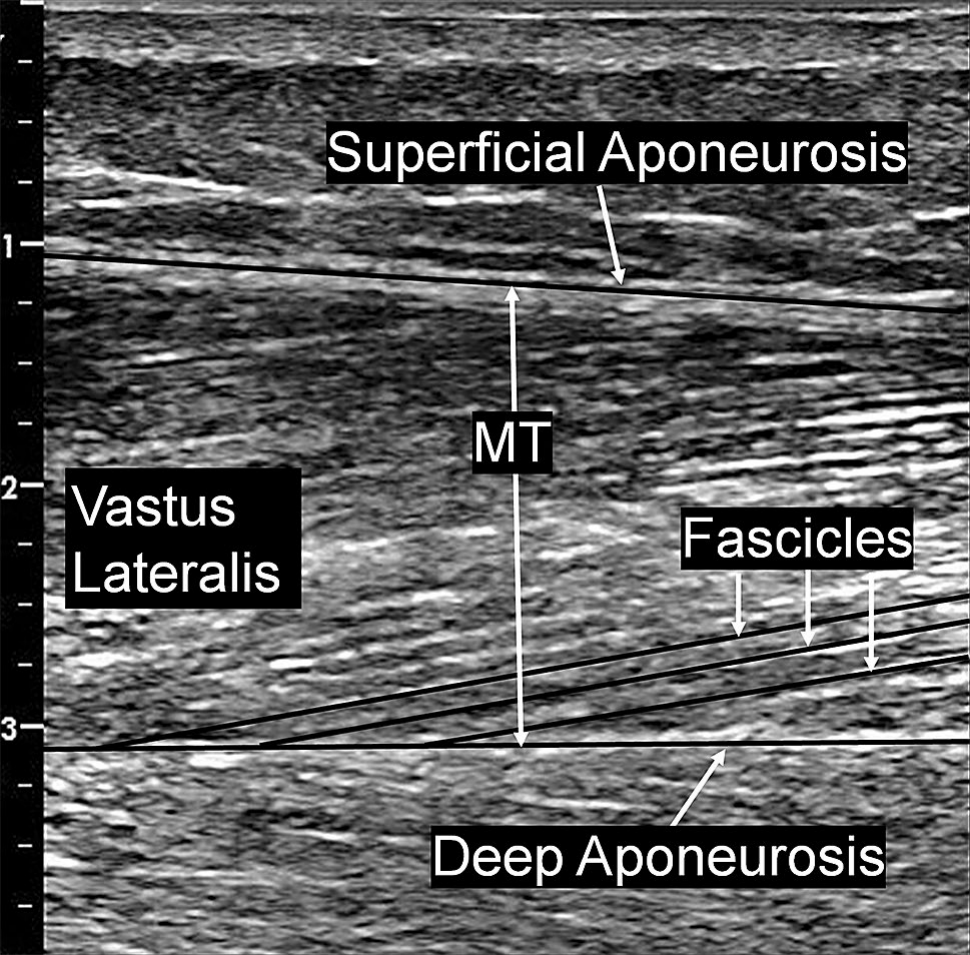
Ultrasound image of vastus lateralis muscle architecture. Muscle thickness (MT) was measured at the centre of the image between superficial and deep aponeuroses, with pennation angle (PA) calculated as the average from three fascicles, and standard trigonometry was used to calculate fascicle length
